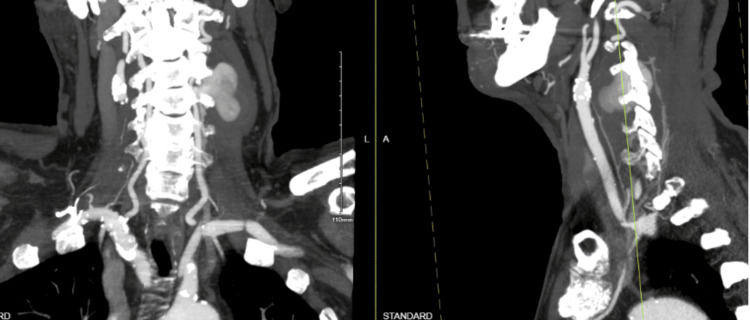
Computed tomography angiography (CTA) of neck CTA of neck demonstrated a paraspinal enhancing mass measuring 2.2 x 3.6 x 4.0 cm (tr x ap x cc) causing 75% narrowing of the left vertebral artery at the level of C2-C3 (2:176) likely representing a pseudoaneurysm with extension into the left intraspinal epidural space and the left paraspinal and displacing the paraspinal muscles.
